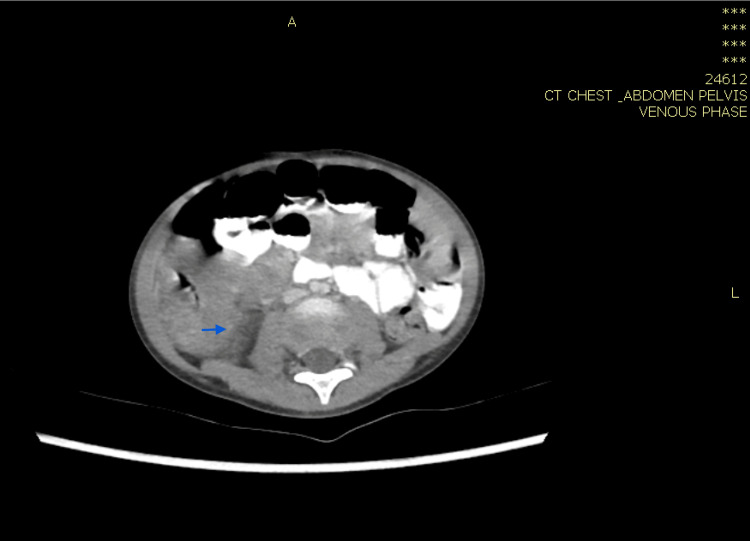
Computed tomography scan of the abdomen with IV contrast showing average liver size and well-defined marginally calcified lesion approximately 1.8 cm in the posterior segment of the right lobe, consistent with calcified granuloma. Healing of the previously noted hepatic abscesses (blue arrow) was observed.
